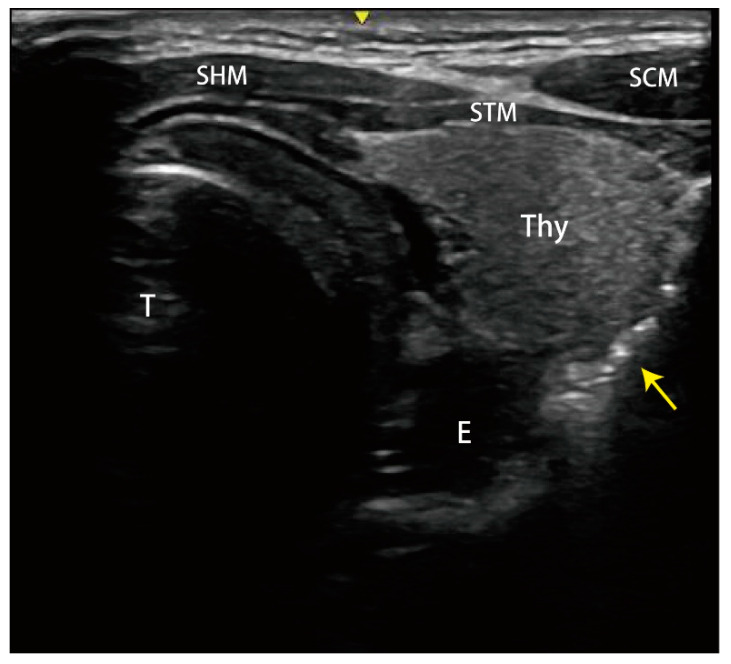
Point-of-care ultrasound found the free air (yellow arrow) in the soft tissue of the neck with ring-down artifacts along the esophageal tract. STM: sternothyroid muscle; SHM: sternohyoid muscle; SCM: sternocleidomastoid muscle; E: esophagus; T: trachea; Thy: thyroid gland.
